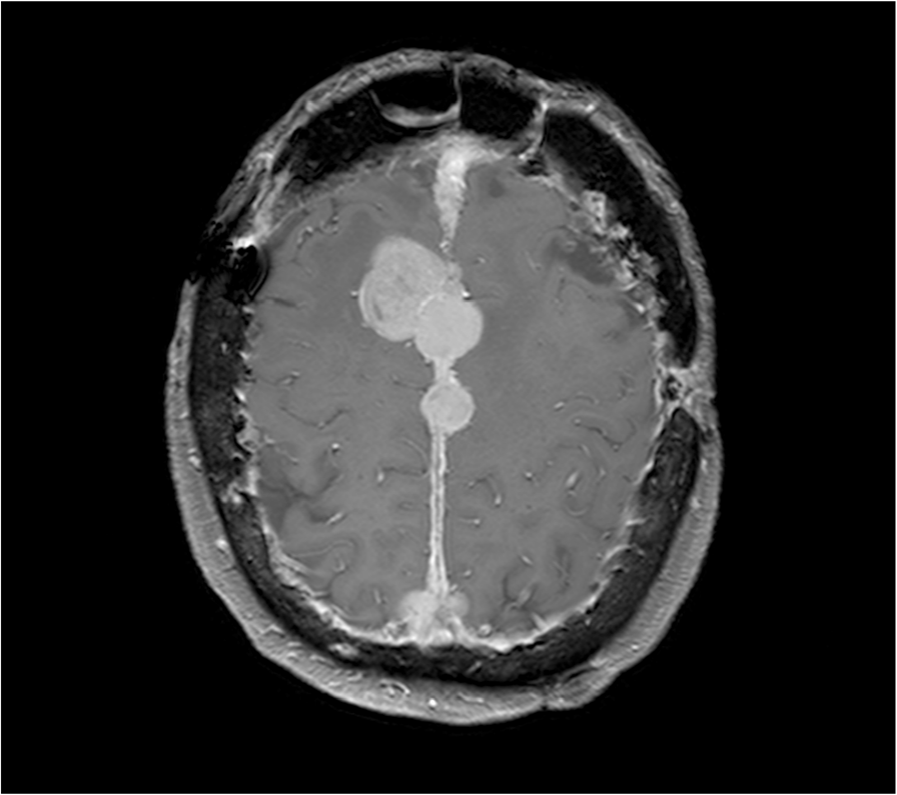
Brain MR with contrast. This T1-weighted image detected multiple meningiomas enhancing intensely and homogeneously after administration of gadolinium.
